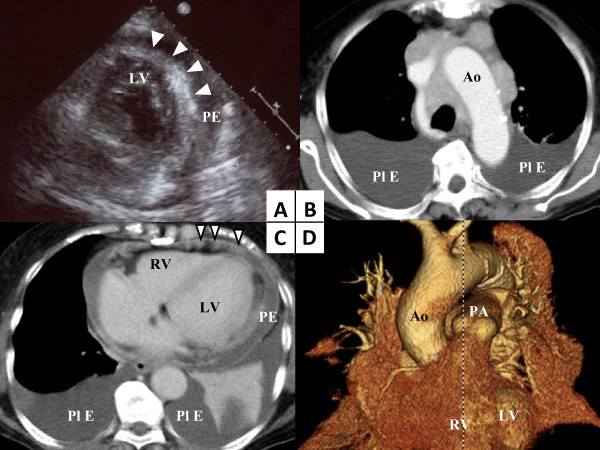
Imaging studies. (A) Echocardiography revealed a high echoic region outside the ventricular wall (arrow head). (B, C) The computed tomography scan on admission revealed a mediastinal mass, pericardial nodules (arrow head), and pericardial and bilateral pleural effusions. (D) Three-dimensional computed tomography reconstruction imaging revealed the rough surface of the epicardium. Ao: aorta; LV: left ventricle; PA: pulmonary artery; PE: pericardial effusion; Pl E: pleural effusion; RV: right ventricle.
